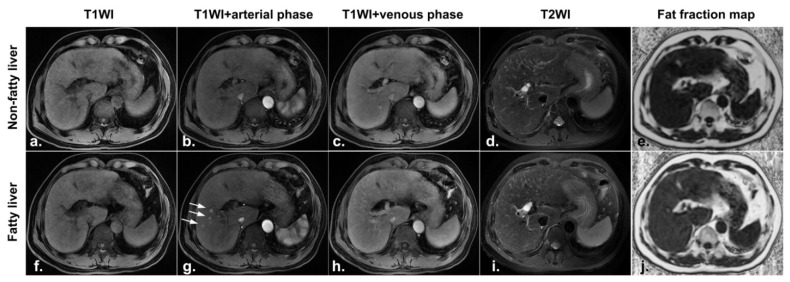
A 51-year-old male patient underwent surgical resection of a small hepatocellular carcinoma. MRI images one month after radiofrequency ablation (a–e): no lesions were found on both T1W (a,f) and T2W (d,i); the fat fraction map showing no fat deposition in the liver (e); contrast-enhanced images showing no abnormal perfusion foci (b,c). MRI images four months after radiofrequency ablation (f–j): a fat fraction map showing new fat deposition in the liver (j); a contrast-enhanced image in the arterial phase showing hyperperfused foci(white arrows) in the liver (g); isointense signals in the portal venous phase (h).
